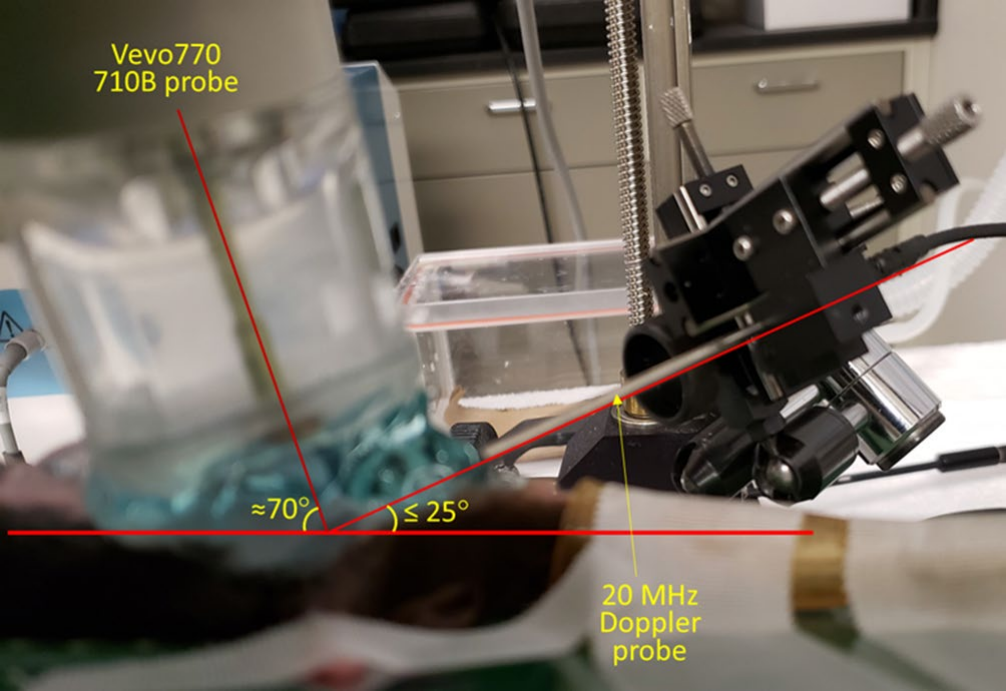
Experimental setup to simultaneously measure ascending aortic Doppler flow velocity using DFVS, left ventricular dimensions and ascending aortic flow velocity using VEVO770 system with 710B probe. Also, shown in the figure are lines representing the horizontal plane, and the angle of orientation of 20 MHz Doppler probe (≤ 25°) and that of the 710B probe (≈70°).
